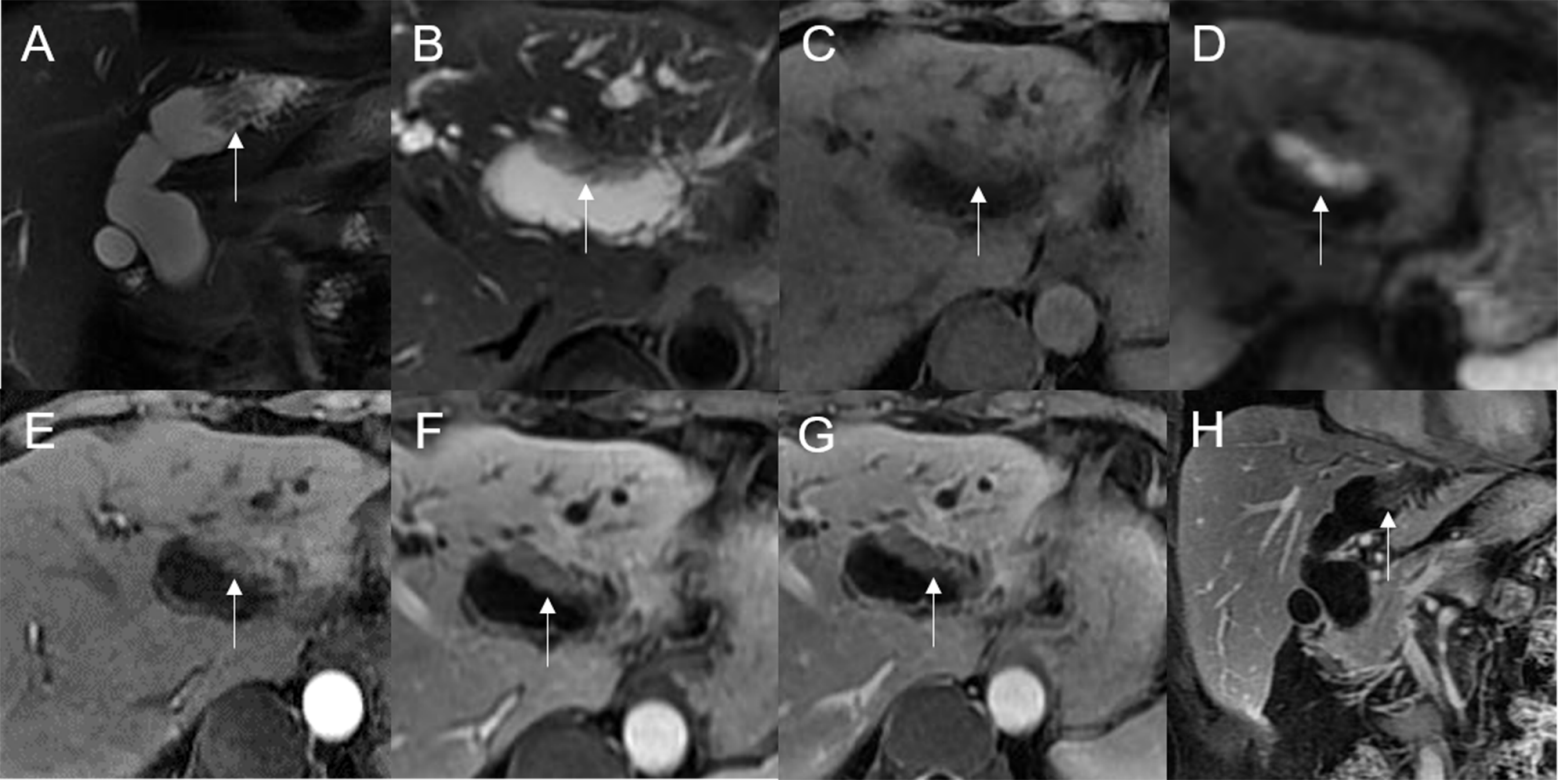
Adenoma in the left hepatic duct. The plaque-like mass (white arrow) shows isointensity on T2WI (A, B), isointensity on T1WI (C), and hyperintensity on DWI (D). The bile duct upstream, downstream, and at the lesion is dilated on coronal T2WI (A). T1-postcontrast arterial phase (E), portal phase (F), and delayed phase (G, H) all show slight enhancement.
